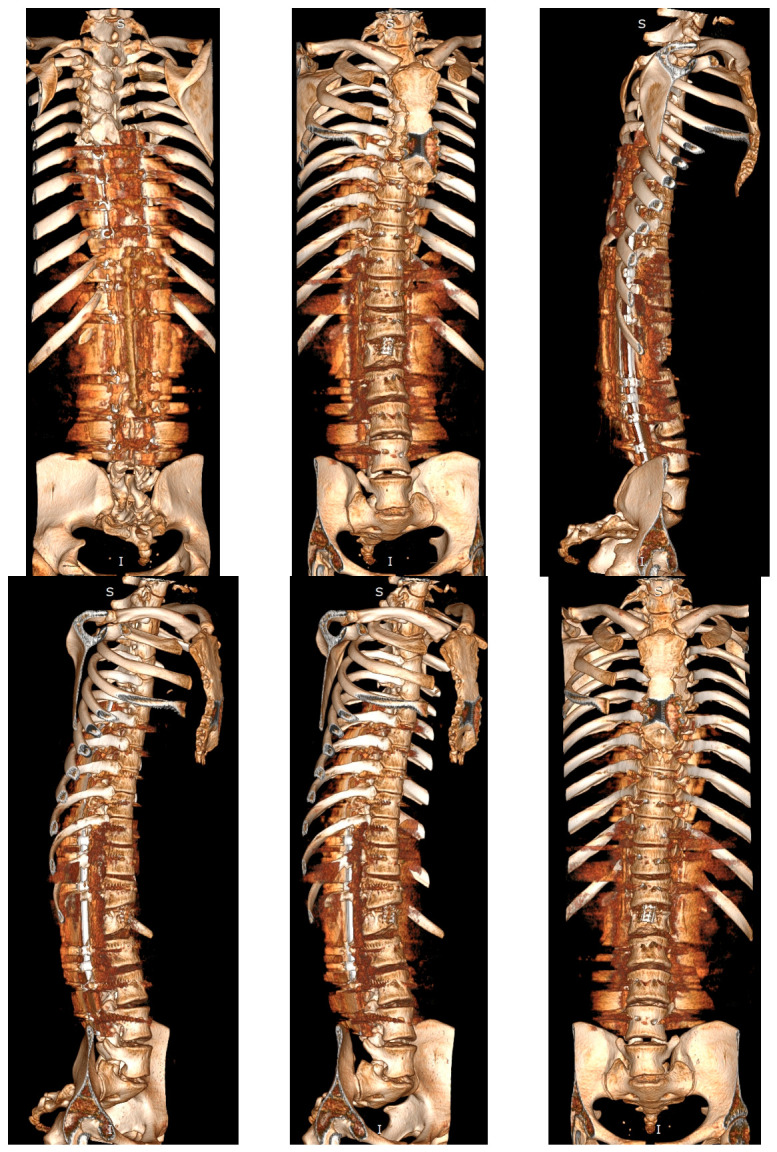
Postoperative 3D-CT of a 16-year-old girl with achondroplasia and congenital severe kyphosis after undergoing surgical treatment at the most recent follow-up and clinical evaluation (2.5 years after surgery).
